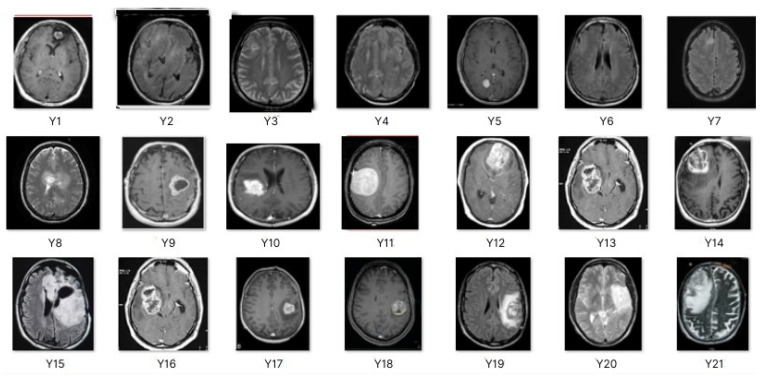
MRI samples Normal Brain MRI (Y1 to Y8) Benign tumor MRI (Y9 to Y15) Malignant tumor MRI (Y16 to Y21).
